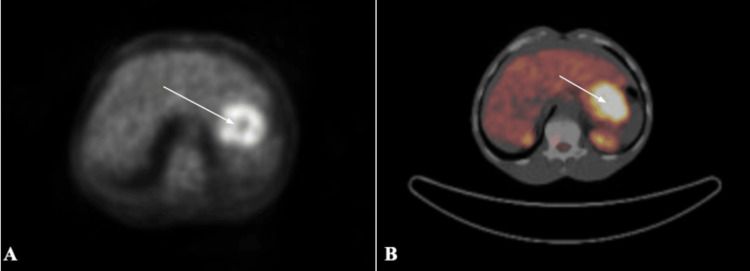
Initial PET/CT (a) CT scan axial view at the level of the abdominal viscera showing increased uptake in the gastric region, (b) F18 FDG PET fused axial imaging showing increased diffuse appearing uptake throughout the gastrum with a max SUV of 7.2. Given this increased uptake in the setting of the patient’s abdominal pain and active malignancy, there was a concern for this being representative of an extracutaneous manifestation of his pcAECTCL in his GI tract. PET/CT: positron emission tomography/computed tomography; F18 FDG PET: fludeoxyglucose-F18 positron emission tomography; SUV: standardized uptake value; pcAECTCL: primary cutaneous aggressive epidermotropic cytotoxic T-cell lymphoma
